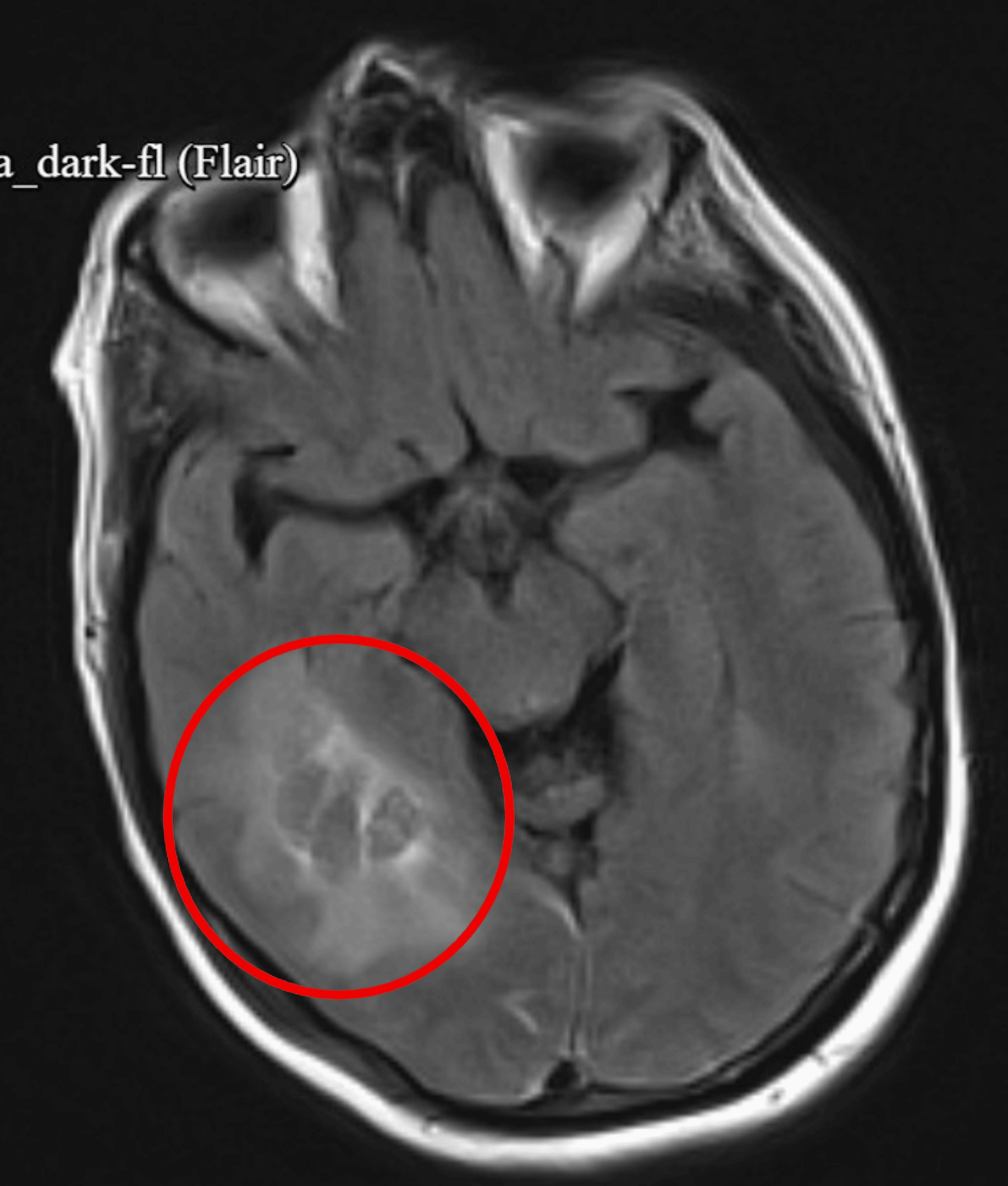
Axial FLAIR brain MRI demonstrates a subacute right temporal intraparenchymal hemorrhage. FLAIR: fluid-attenuated inversion recovery, MRI: magnetic resonance imaging
